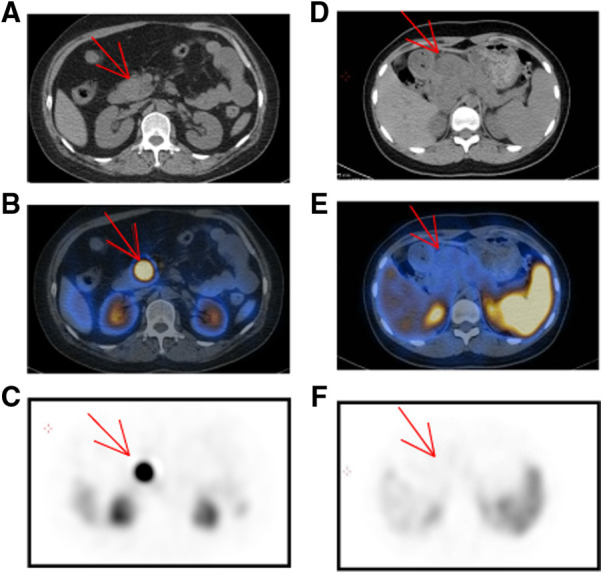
Characteristics of pNET and SPT in ^99m^Tc-HYNIC-TOC PET/CT. (A–C) A 47-year-old woman was suspected of pNET in the pancreatic head. Upon noncontrast CT examination, a predominant solid and regular shape mass was observed [(A), arrow]. In the ^99m^Tc-HYNIC-TOC PET/CT fusion images, there was intense activity in the pancreatic head [(B), arrow]. (D–F) SPT in a 14-year-old girl with abdominal pain. Upon unenhanced CT examination, a 5.8 × 5.2 cm mass was presented in the pancreatic head with an ill-defined margin and low density [(D), arrow]. In the ^99m^Tc-HYNIC-TOC PET/CT fusion images, there was no increased activity in the corresponding location with CT and peripheral tissue was apparently squeezed by the pancreatic mass. pNET, pancreatic neuroendocrine tumor; CT, computed tomography; PET, positron emission tomography; SPT, solid pseudopapillary tumor.
